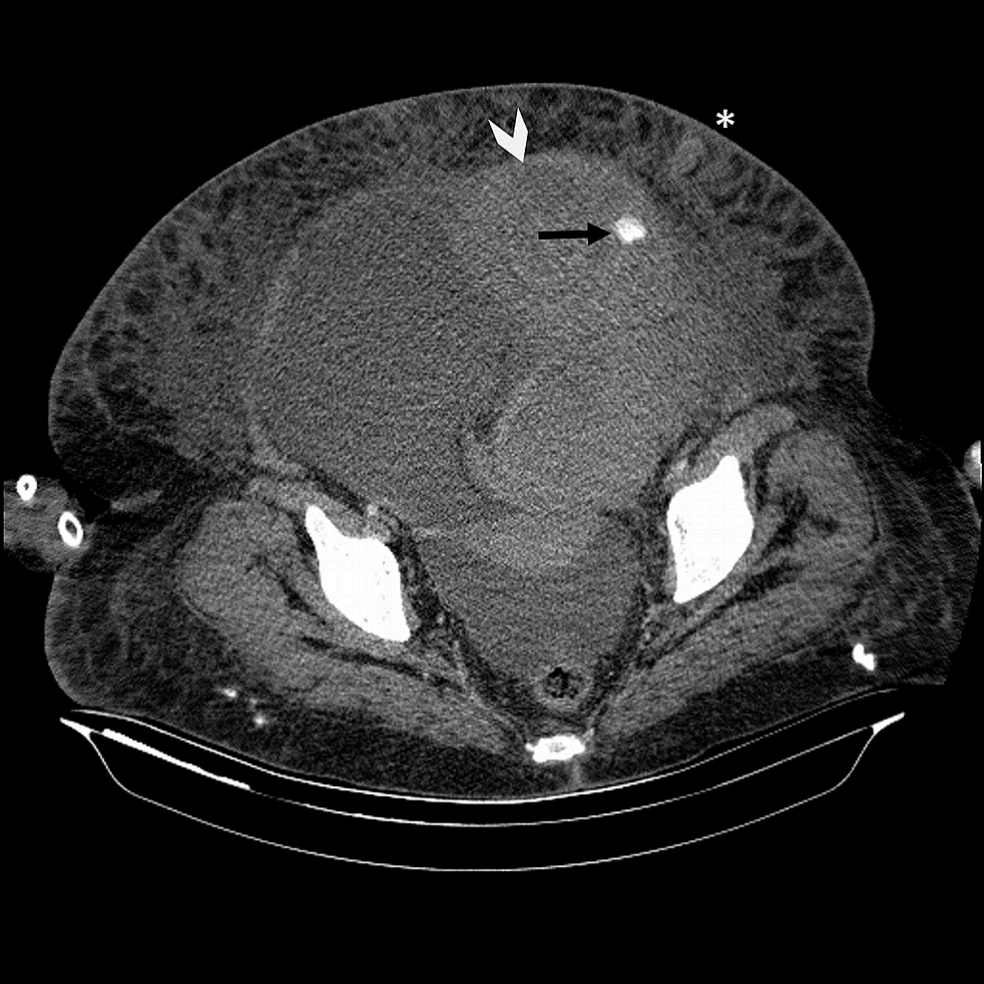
CT findings of rectus hematoma. Black arrow: active contrast leak in the inferior epigastric artery area. Arrowhead: giant hematoma. Asteriks: paracentesis site. CT, computed tomography
